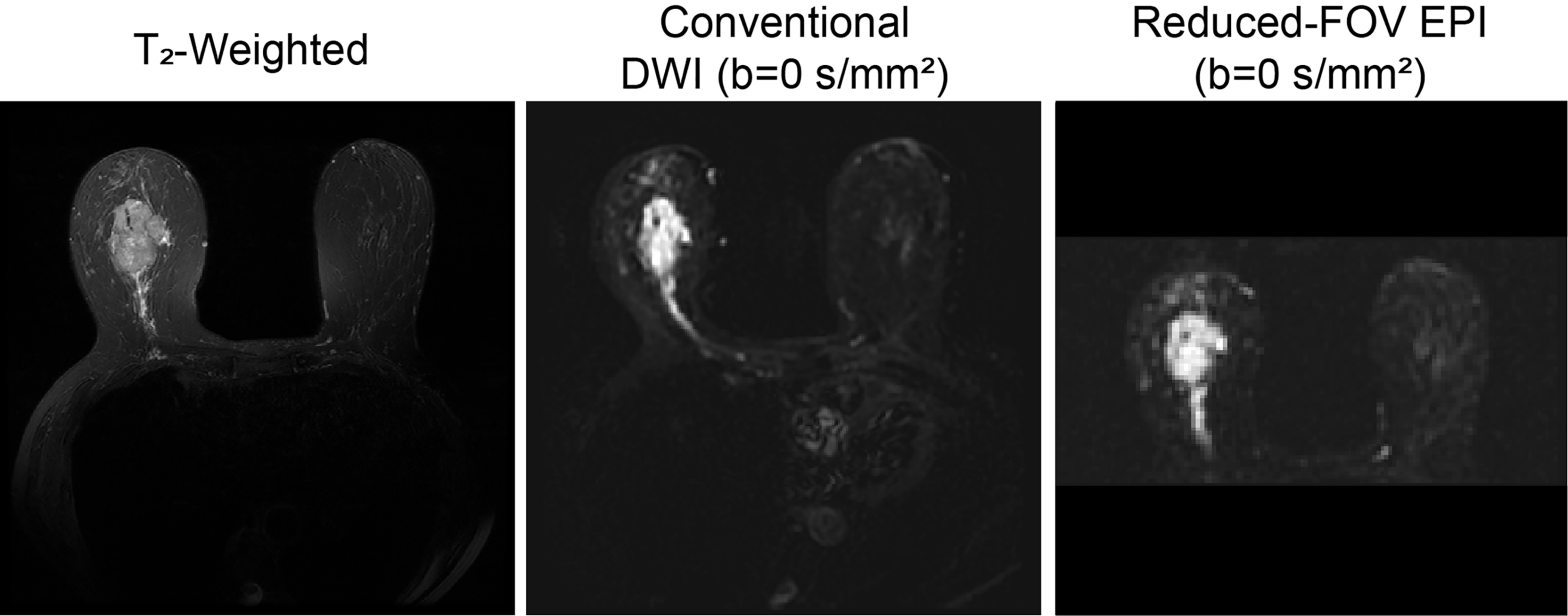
Reduced FOV EPI in a 63-year-old patient with invasive ductal carcinoma. T_2_-weighted, conventional DWI (b=0 s/mm^2^), full FOV EPI (b=0 s/mm^2^), and reduced FOV EPI (50% phase field of view) (b=0 s/mm^2^ acquired at 3T) images are shown. Reduction of percent phase encoding direction to 50% reduces geometric distortions caused by B_0_-inhomogeneity, especially in the nipple region (100).
